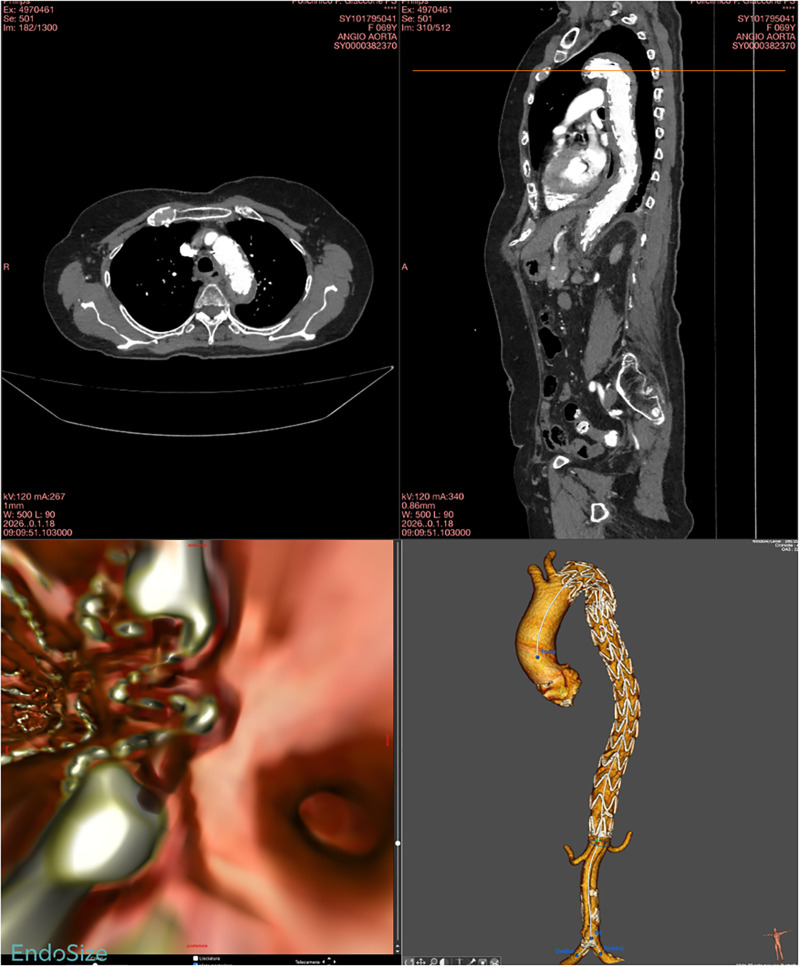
Follow-up CT scan and 3D reconstruction of TEVAR using EndoSize software.
